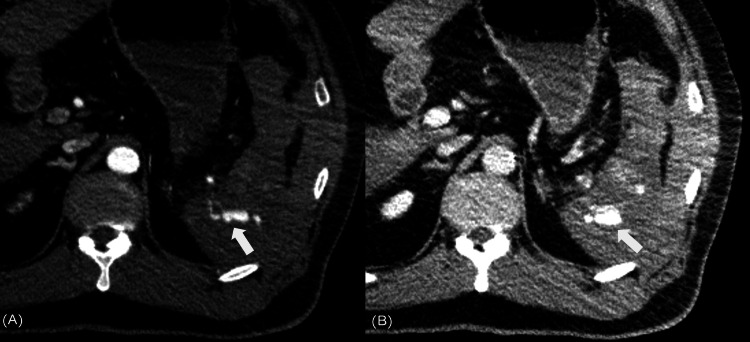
Whole body CT performed immediately before REBOA balloon deflated (A) Contrast-enhanced computed tomography arterial phase indicating extravasation (arrow) outside the spleen. (B) In the venous phase, extravasation (arrow) appears enlarged, indicating active bleeding. CT, computed tomography; REBOA, resuscitative endovascular balloon occlusion of the aorta
